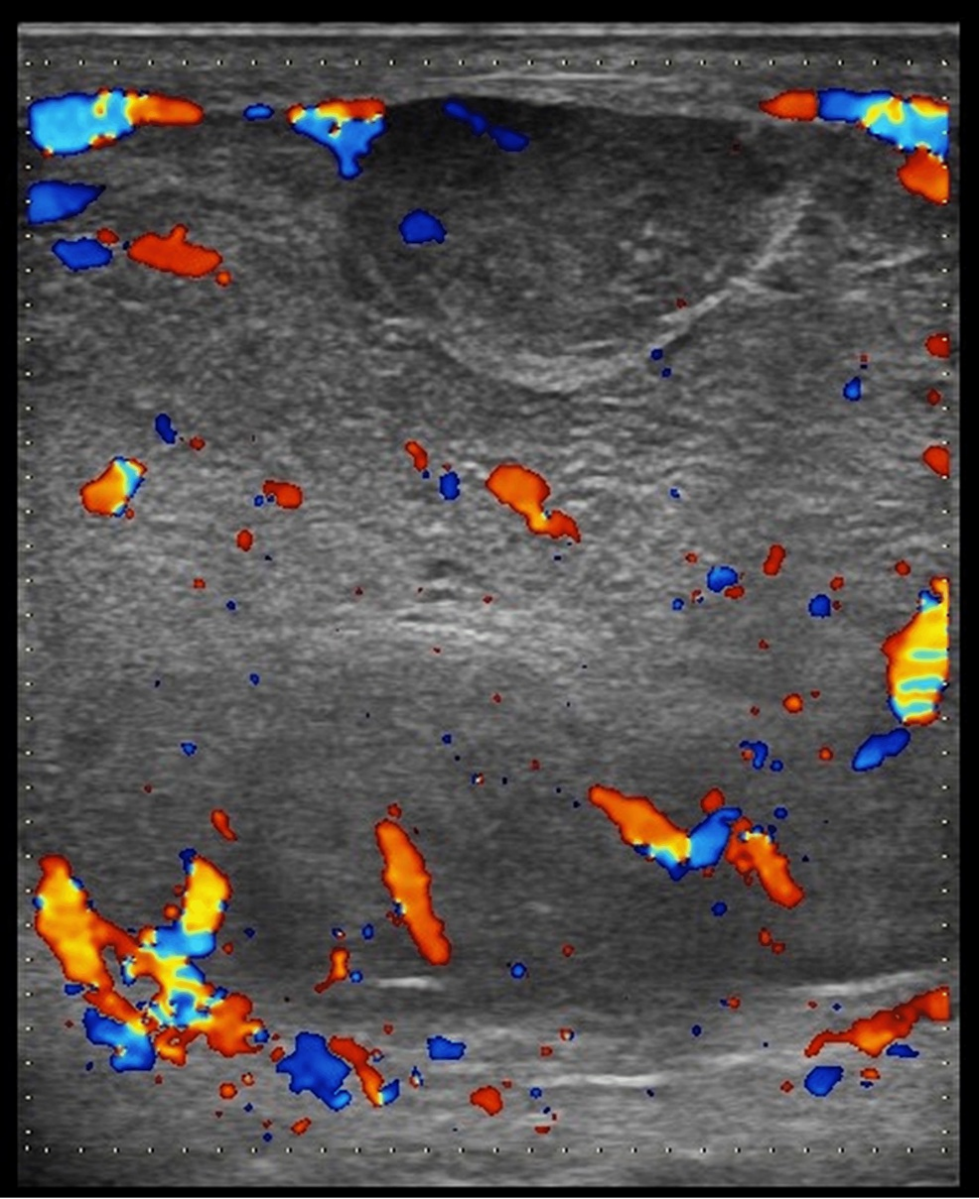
Doppler imaging of the right testis showing increased vascularity in the peripheral parts.
